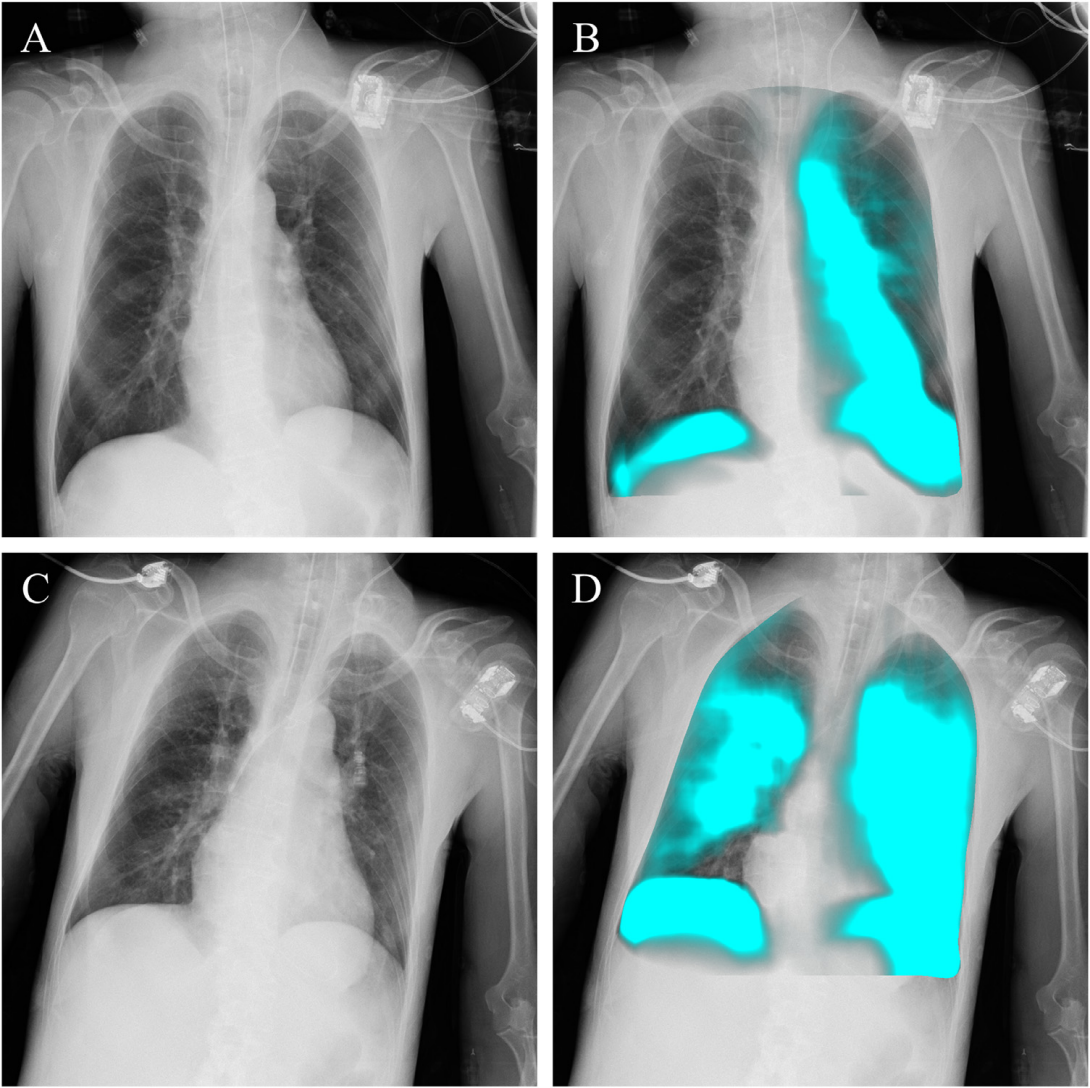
Dynamic chest radiography in a patient with suspected mucus plugging. Dynamic chest radiography during a wheezing episode suspected to be caused by mucus plugging shows the original image (A) and the PL-MODE image (B). In the PL-MODE image, reduced pixel value changes in the right lung were observed, suggesting impaired ventilation. After bronchoscopic suction, clinical improvement was achieved. The follow-up dynamic chest radiography, including the original image (C) and PL-MODE image (D), demonstrated improved ventilation distribution.
